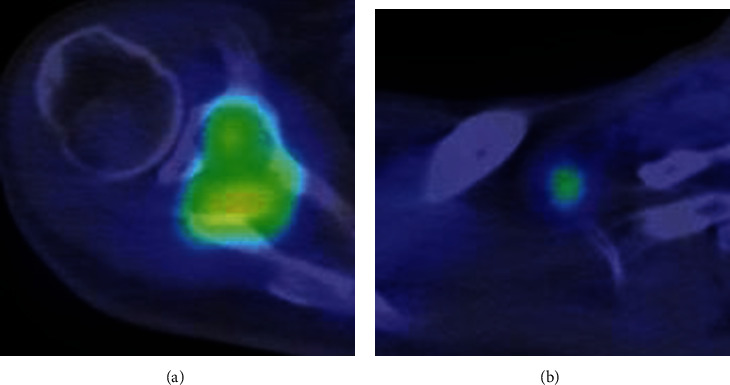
^18^F-FDG-PET/CT scan at first presentation. PET/CT fusion image shows increased FDG uptake in (a) the scapular tumor (SUV max 4.16) and (b) axillary lymph node (SUV max 2.8).
